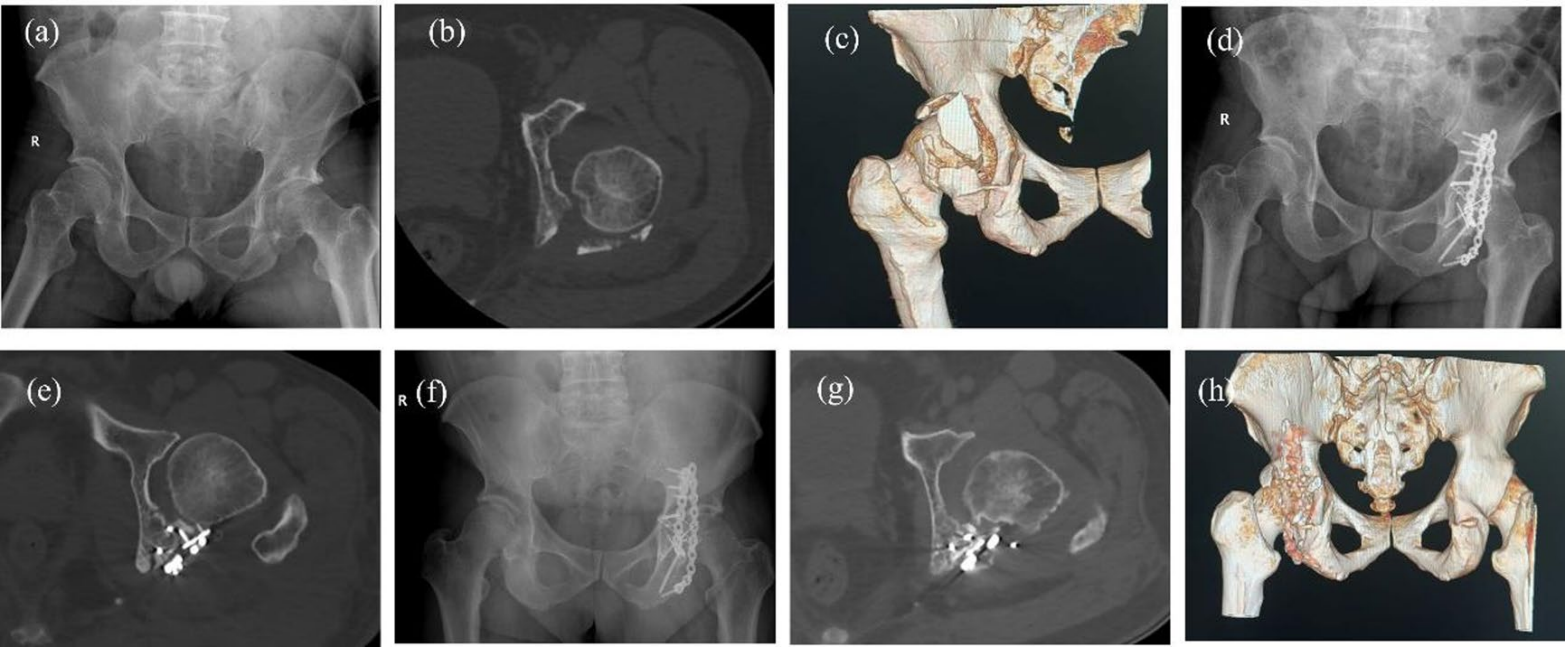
A representative case of PTOA following posterior wall acetabular fracture(a-h). (a) A 55-year-old man sustained a traffic accident resulting in a left posterior wall acetabular fracture. (b, c) Preoperative CT cross-section and three-dimensional (3D) CT examination are shown. (d) The patient underwent posterior wall acetabular fixation with a locking plate. (e) Postoperative CT cross-section revealed partial joint surface compression changes. (f) One month post-surgery, no significant joint degeneration was observed. (g, h) Seven months post-surgery, CT cross-section and 3D CT examination showed joint surface sclerosis and surrounding osteophyte formation, indicating the development of PTOA.
